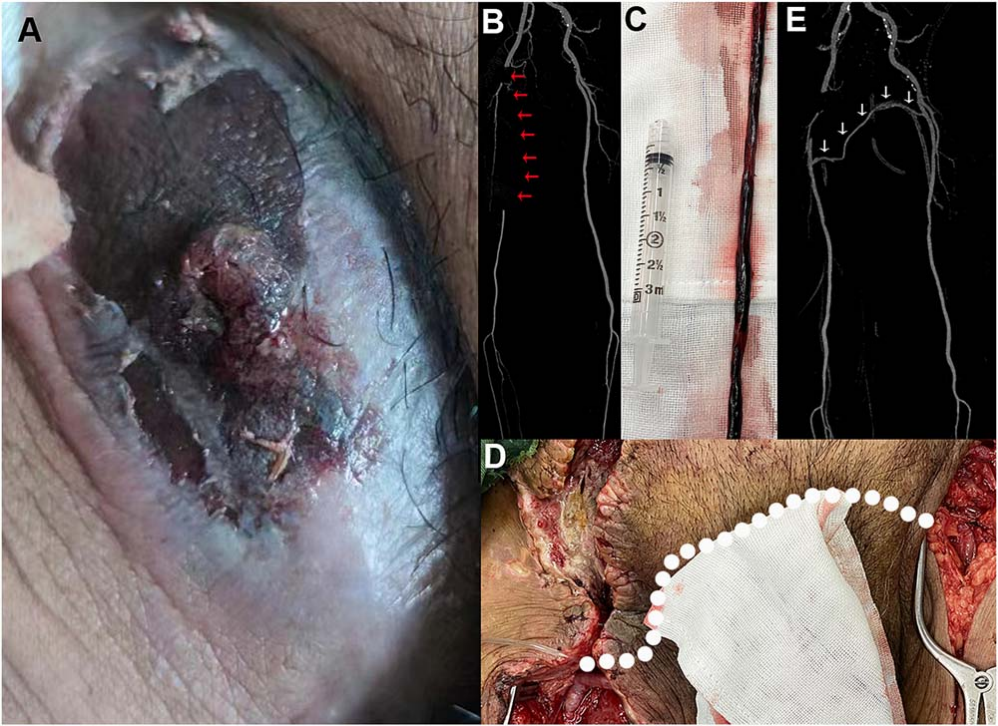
Representative images of femoral artery pseudoaneurysm. (A) A preoperative pulsatile hematoma in the right groin. (B) CT image with the red arrows pointing to the obstructive superficial femoral artery. (C) The removed arterial thrombosis. (D) Intraoperative bypass of left-to-right femoral artery; white dashed line showing the autologous vein trajectory. (E) Postoperative computed tomography angiography image showing the autologous vessel (white arrows) and the right extremity demonstrating patent arterial flow.
